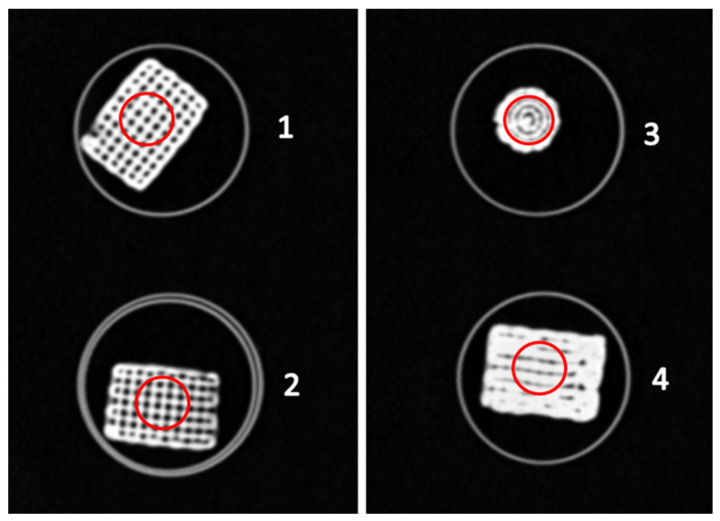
Examples of the CT-scanned scaffolds and selected ROIs for each of the scaffolds. Scaffold #1, Scaffold #2, Scaffold #3, and Scaffold #4.
